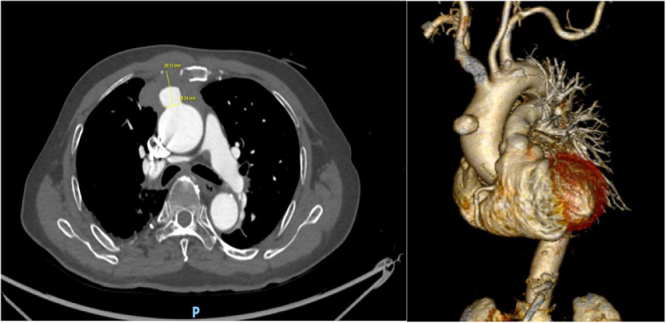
Contrast CT scan showing a focal saccular aneurysm of the anterior wall of the ascending aorta with peripheral enhancement, adjacent periaortic inflammatory changes, and sternal erosion – features suggestive of an infectious aneurysm.
